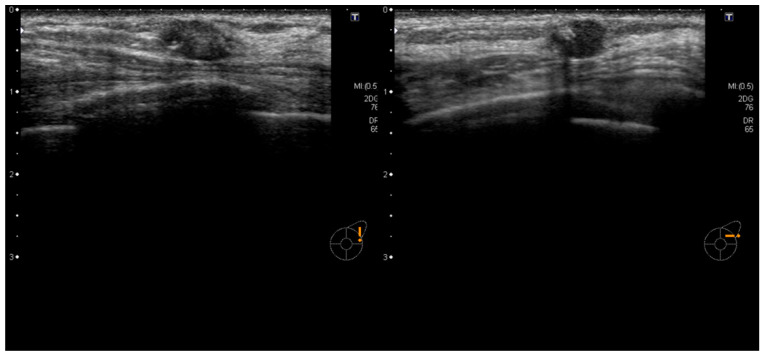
Ultrasound examination revealed a well-defined, oval-shaped tumor measuring 9 mm in the upper outer quadrant of the left breast. Fibroadenoma was suspected, and a plan was made for follow-up observation using ultrasound and mammography.
